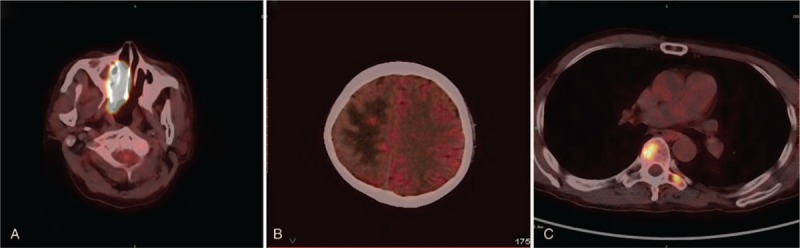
Positron emission tomography CT performed after biopsy revealed a hypermetabolic mass involving the (A) right maxillary sinus, (B) right frontal lobe, and (C) transverse process and T6 vertebra.
